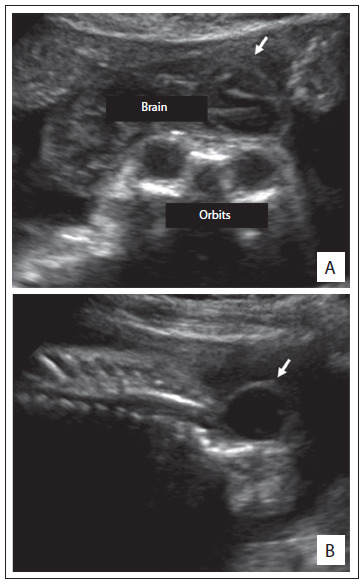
Ultrasound findings from a fetus (case 7) still in at the stage of exencephaly (A, see arrows) and with myelomeningocele (B).
